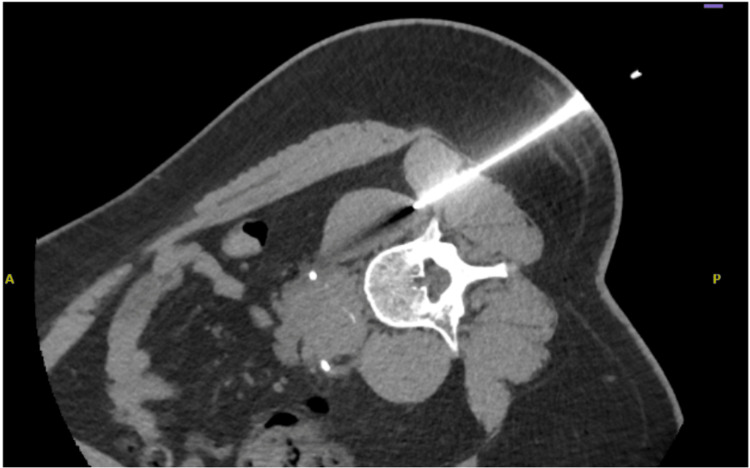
CT-guided biopsy of the retroperitoneal mass. Axial CT image demonstrating percutaneous CT-guided biopsy of the retroperitoneal periaortic soft-tissue mass, with the biopsy needle positioned within the lesion for tissue sampling. CT: Computed tomography
